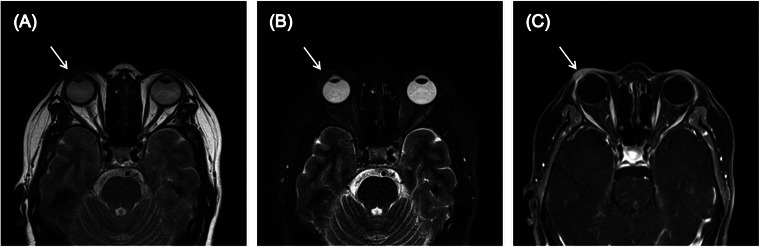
Magnetic resonance imaging of sebaceous carcinoma. (A) The nodular mass had iso‐intensity on T1‐weighted images. (B) The nodular mass had iso‐intensity on T2‐weighted images. (C) The nodular mass had obvious enhancement on the contrast‐enhanced MRI.
